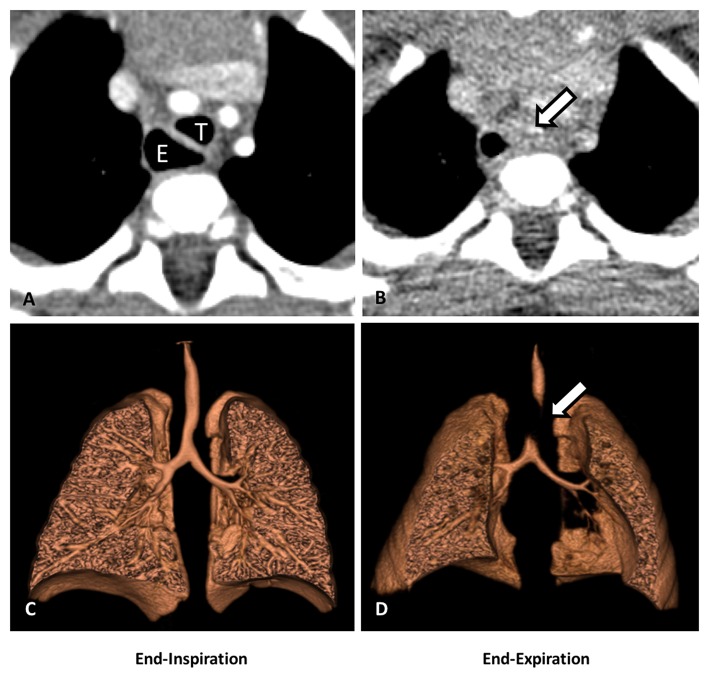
Dynamic airway CT scan with 3-D reconstruction. (A) CT scan (cross-sectional view): partial collapse of the mid trachea (T) and dilated esophageal dilation (E) at end-inspiration. (B) CT (cross-sectional view): complete collapse of the mid trachea (arrow) at end-expiration. (C) 3-D reconstruction of the airways: partial collapse of the mid trachea at end-inspiration. (D) 3-D reconstruction of the airways: complete collapse of the mid trachea at end-expiration (arrow).
